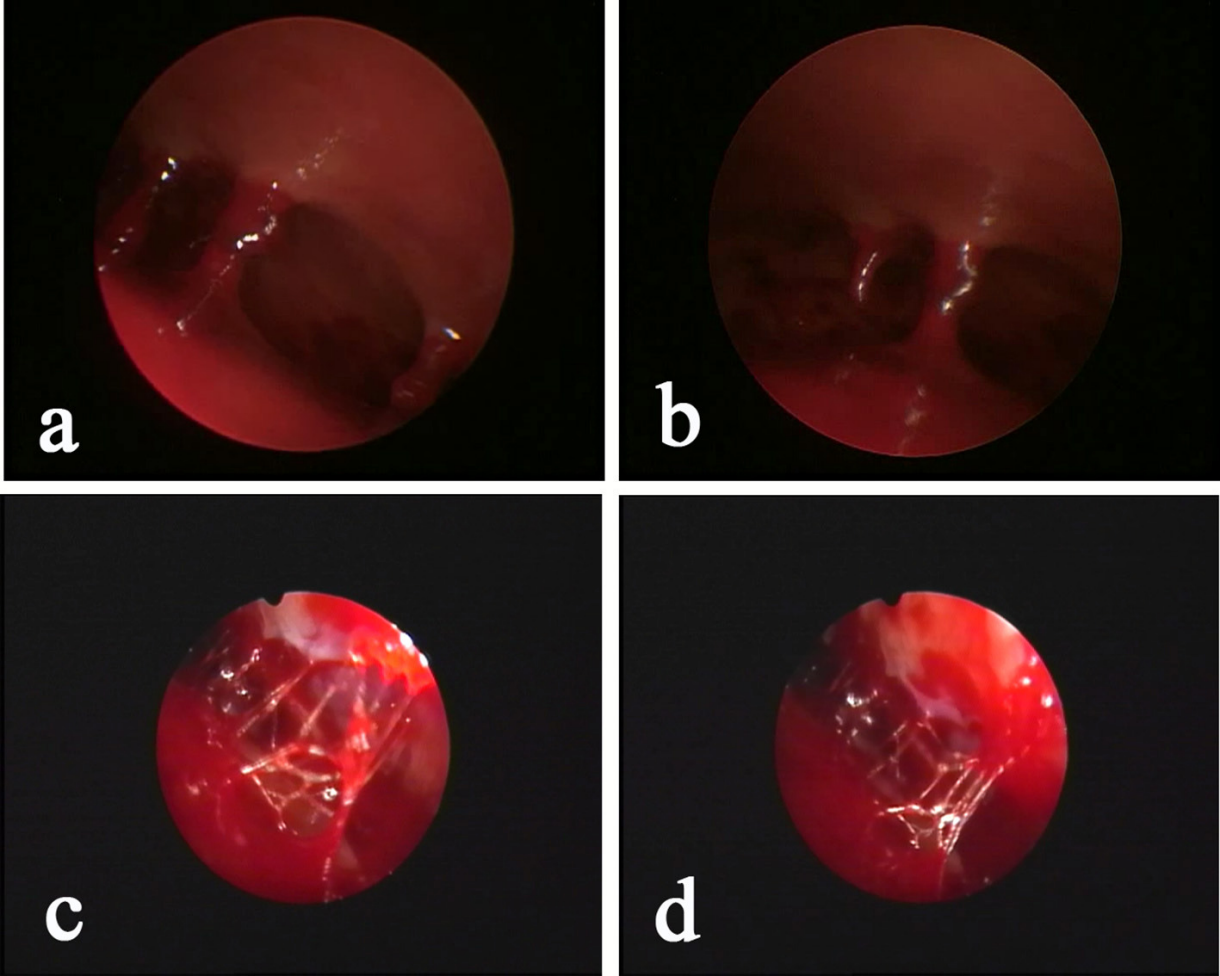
Neuroendoscopic observation of the hematoma with “separation,” as determined by pre-operative CT, shows that the “separation” is mainly a separation lock column (a,b) or separation strip (c,d) instead of a real closed septum. The relative separation is sufficient to block the blood clot in the chamber. Conventional BHD is not sufficient to completely drain the hematoma, but INET is able to remove the separation strip or separation lock column and visually clean the old blood clots.
